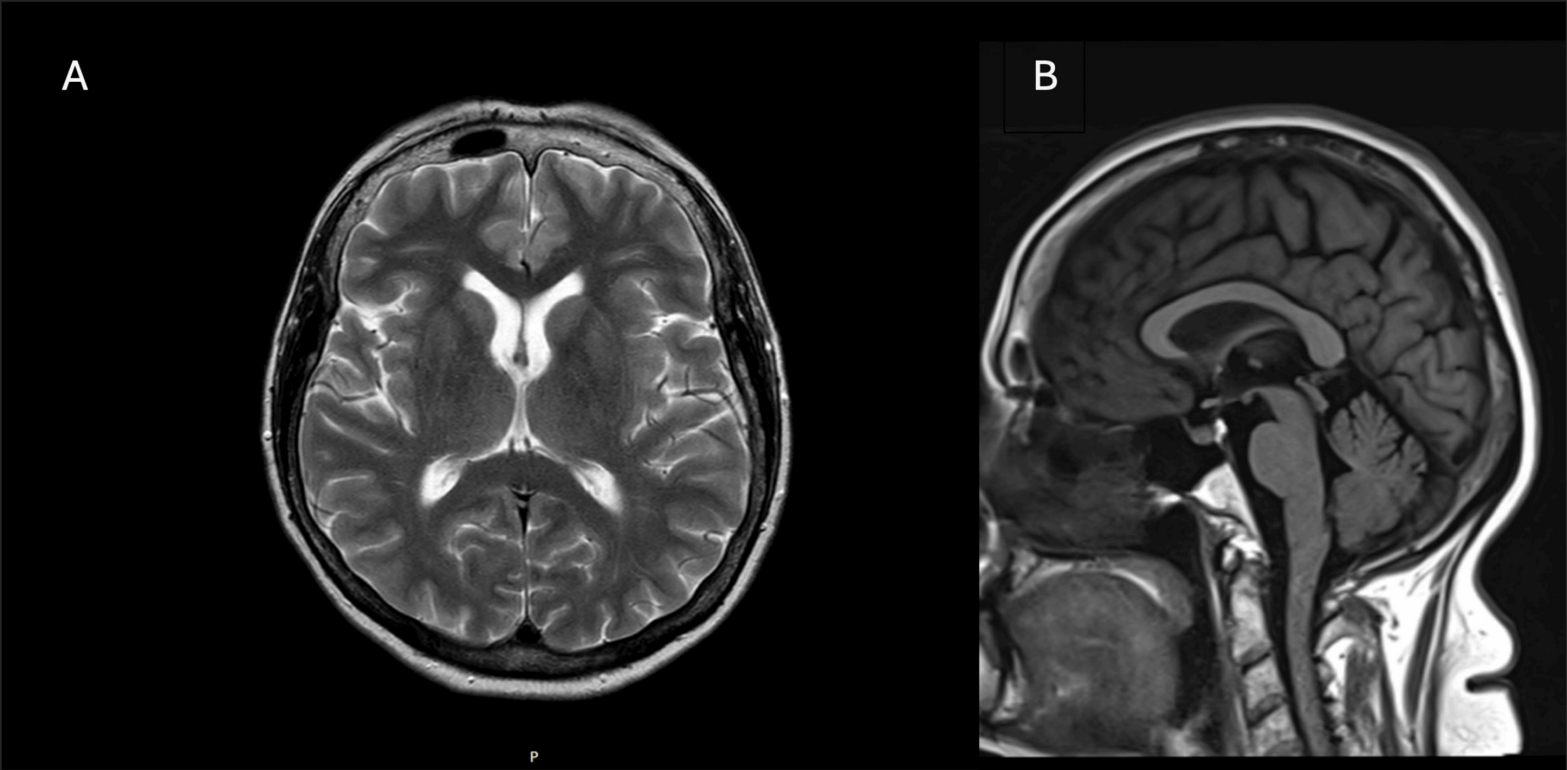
MRI brain demonstrating no abnormalities (A) Axial T2-weighted view and (B) T1-weighted sagittal view. MRI: Magnetic resonance imaging.
